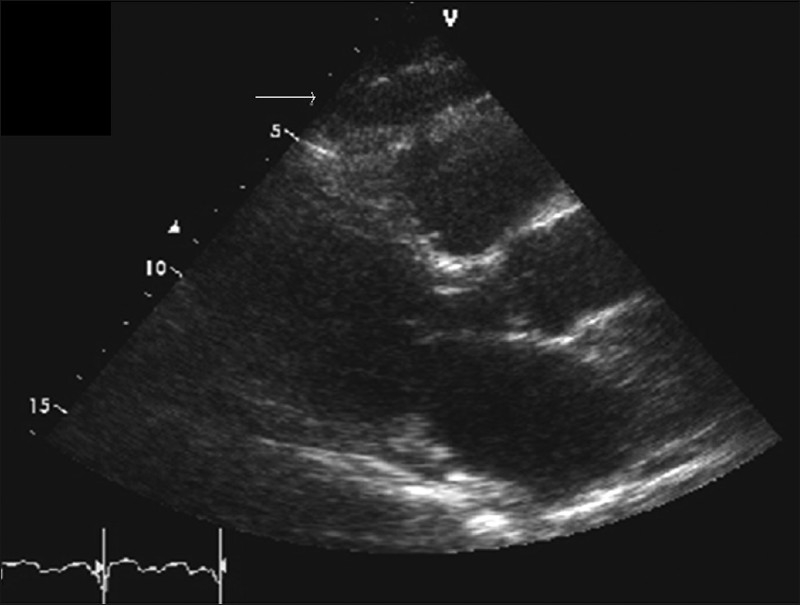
Long-axis parasternal view from transthoracic echocardiogram. The arrow points to the epicardial fat over the free wall of the right ventricle, appearing as the echo-free space between the outer layer of the myocardial wall and the visceral layer of the pericardium
